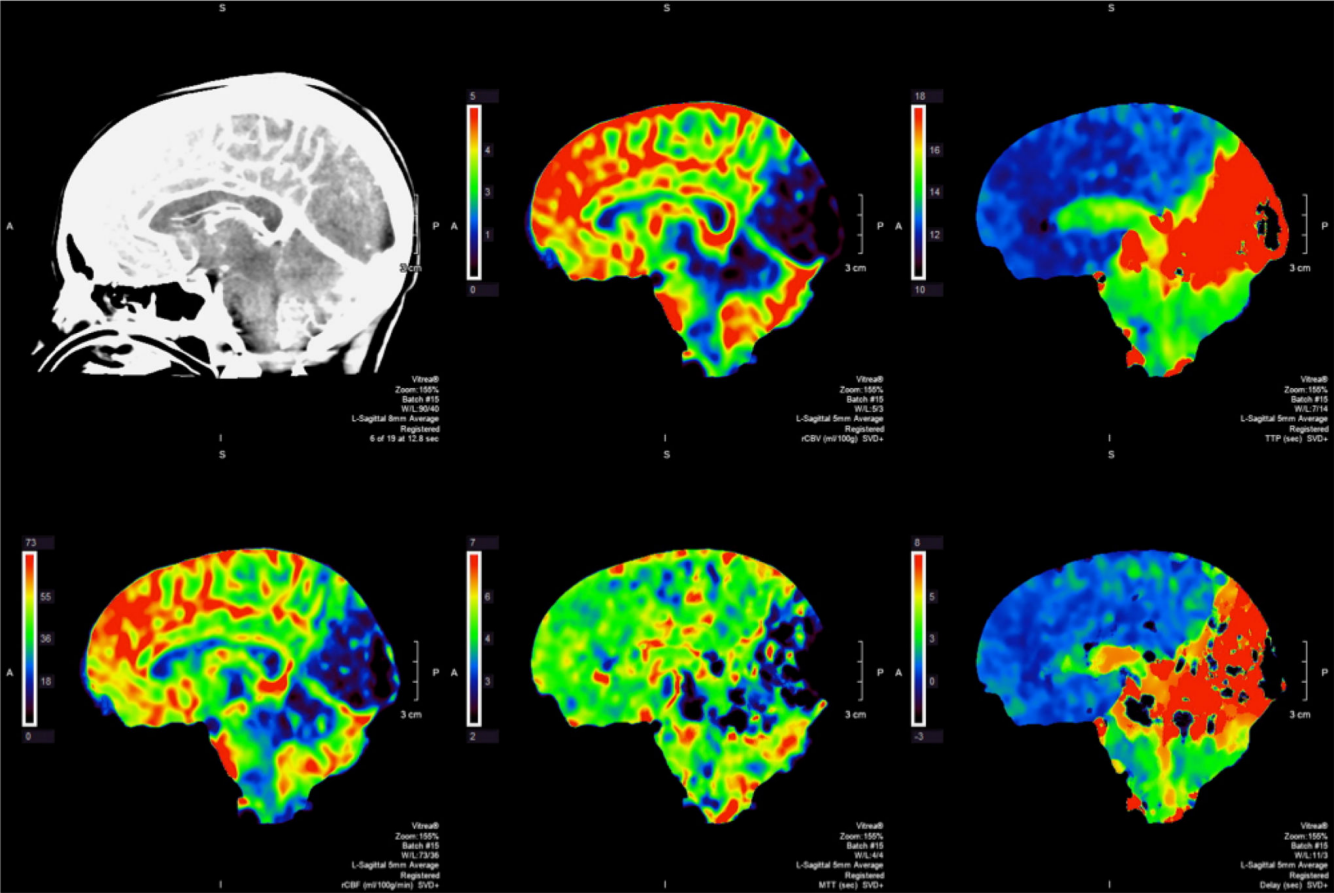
CT Stroke Protocol with perfusion imaging demonstrating primary involvement of occipital lobe, cerebellum, and brainstem. Perfusion imaging reflecting rCBV, TTP, rCBF, MTT, and delay (from top-left to bottom-right).
